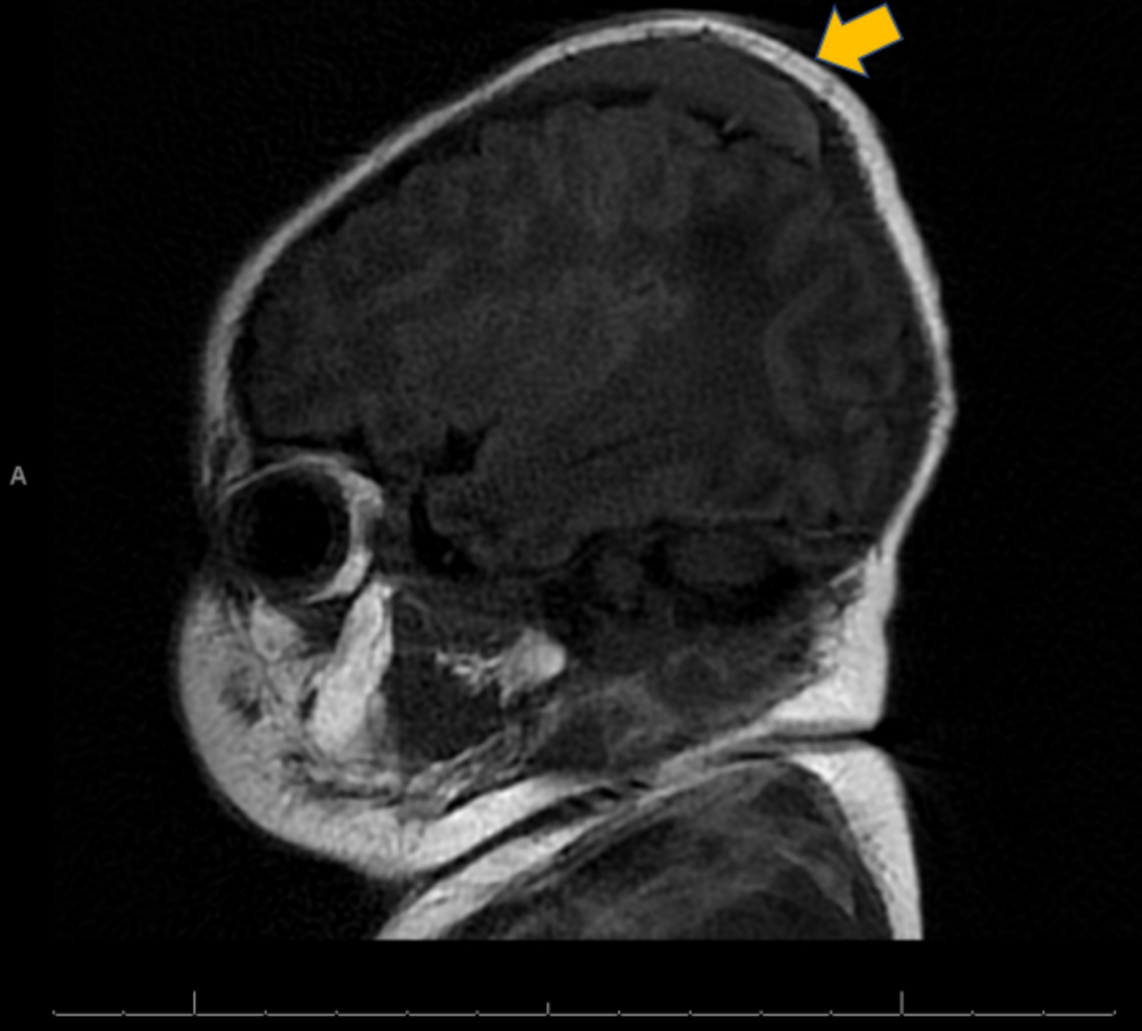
Non-contrast MRI: Left Sagittal View A non-contrast MRI in a left sagittal view showing the presence of a cephalohematoma with no intracranial lesion identified.
